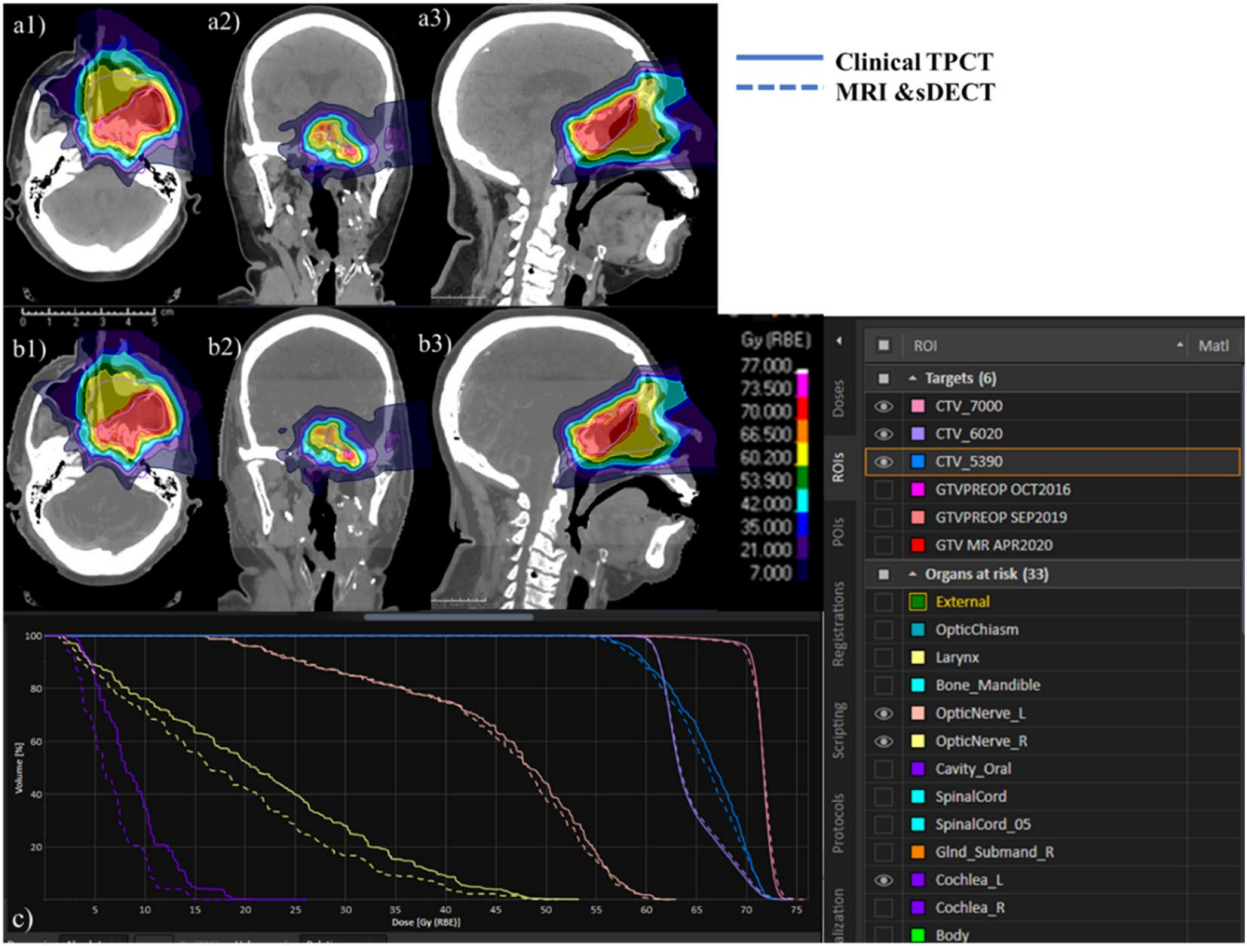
Comparison of dose distribution and DVH between treatment plan using clinical TPCT and MRI and sDECT model (Model D), (a1–a3) shows the dose distribution of treatment plan using clinical TPCT in axial, coronal and sagittal views, (b1–b3) shows the dose distribution of treatment plan using MRI and sDECT method (Model D), (c) shows the cumulative DVH where the solid line are the clinical TPCT based and dashed line are the MRI and sDECT (Model D) based.
